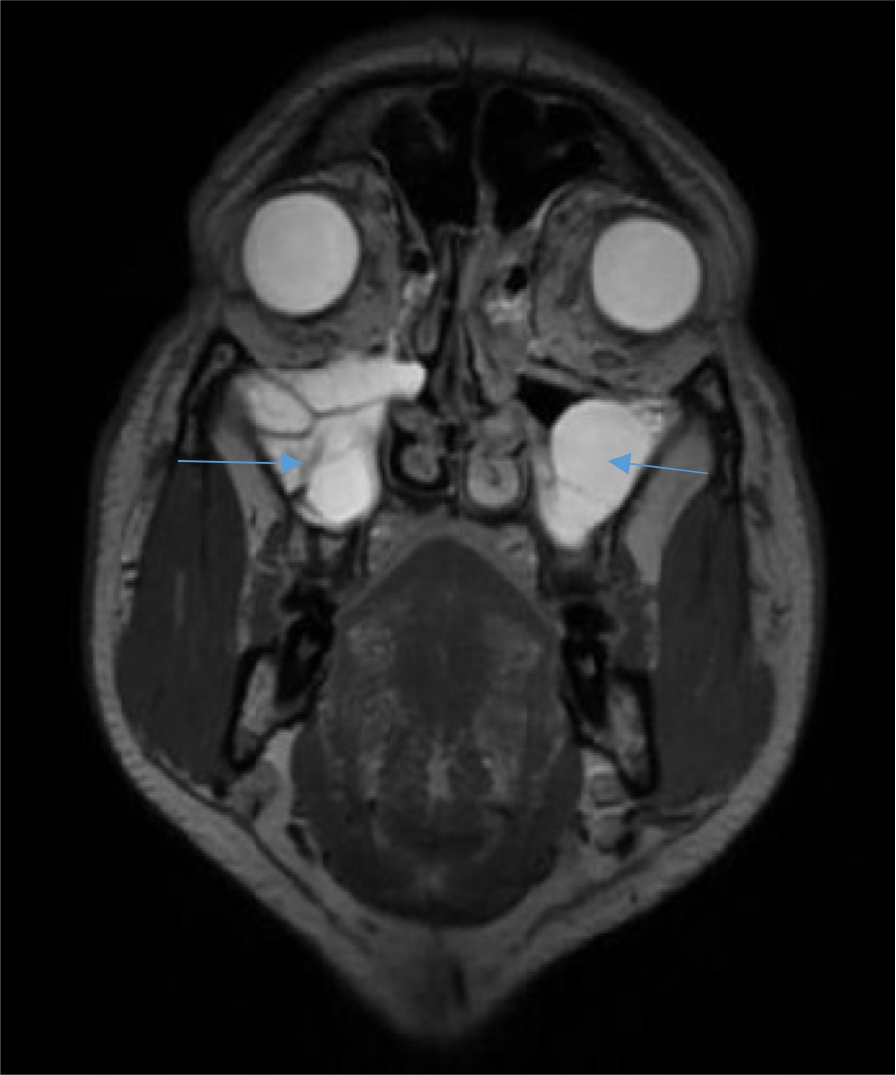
Coronal section of T2-weighted image of a plain MRI of the paranasal sinuses in a patient with sinonasal mucormycosis showing T2 hyperintense mucosal thickening in bilateral maxillary sinuses (blue arrows)
